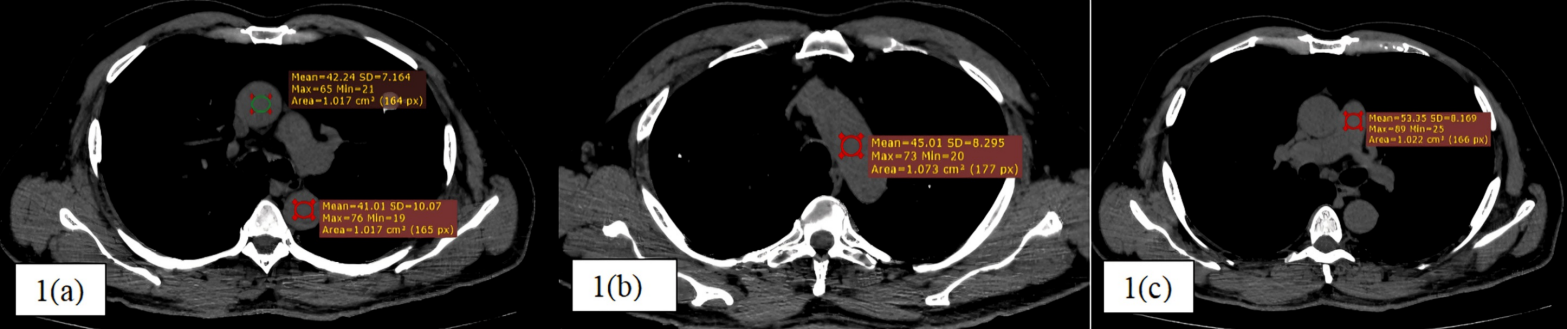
Non-contrast CT thorax-mediastinal window of a 56-year-old non-anemic male patient (serum hemoglobin 15.2 g/dl) showing the circular regions of interest (ROIs) of approximately 1 cm² in standardized anatomical sites, the ascending and descending thoracic aorta (a), aortic arch (b), and main pulmonary artery (c) with mean HU as +42.2, +41.0, +45.0, and +53.3, respectively, which falls within normal limits of non-anemic patients
